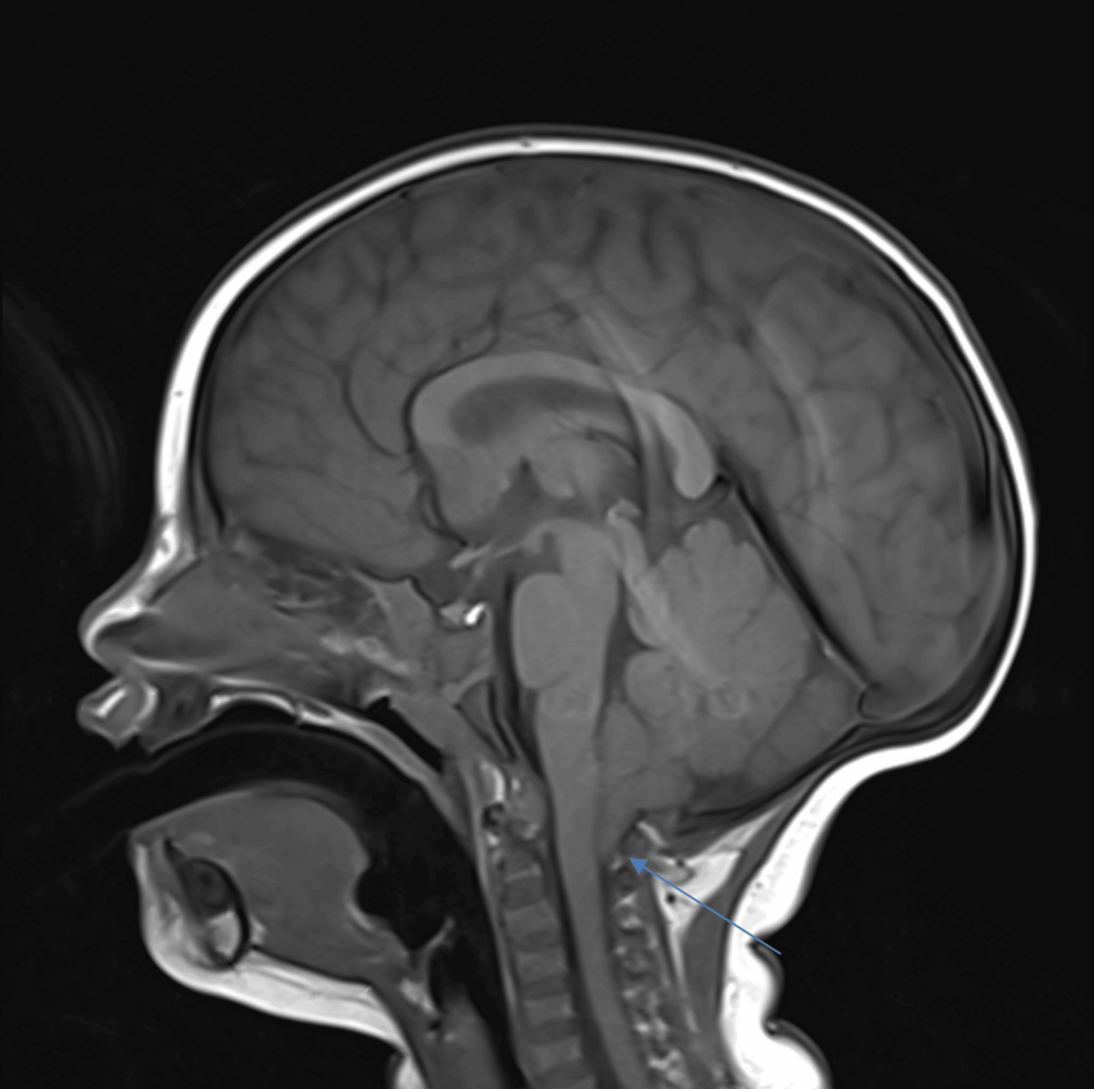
Sagittal T1 MRI imaging of the proband’s sister prior to surgical decompression. The blue arrow indicates cerebellar tonsils extending 10 mm below the foramen magnum.
